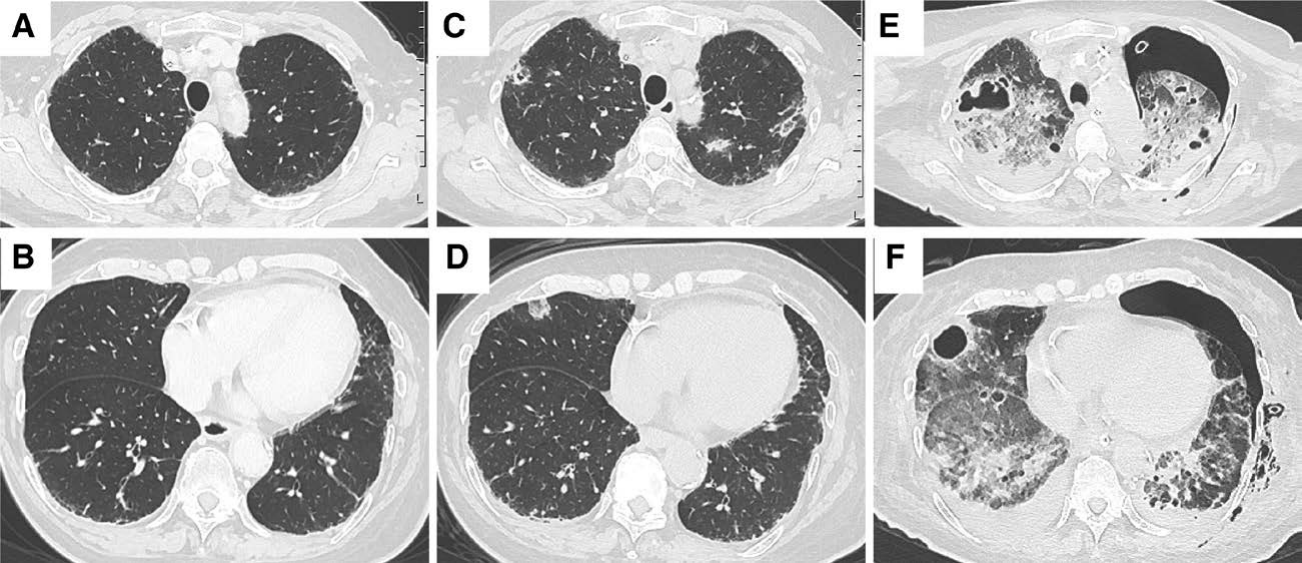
Chest CT time course of the case. (A, B) Recorded before this admission. No remarkable abnormalities were found in bilateral lung field. (C, D) Recorded on day one. Multiple ground-glass opacity and nodular shadow was seen in bilateral lung field. (E, F) Recorded on day 9. Appearance of multiple cavitary lesions and infiltrative shadow in bilateral lung field. CT = computed tomography.
